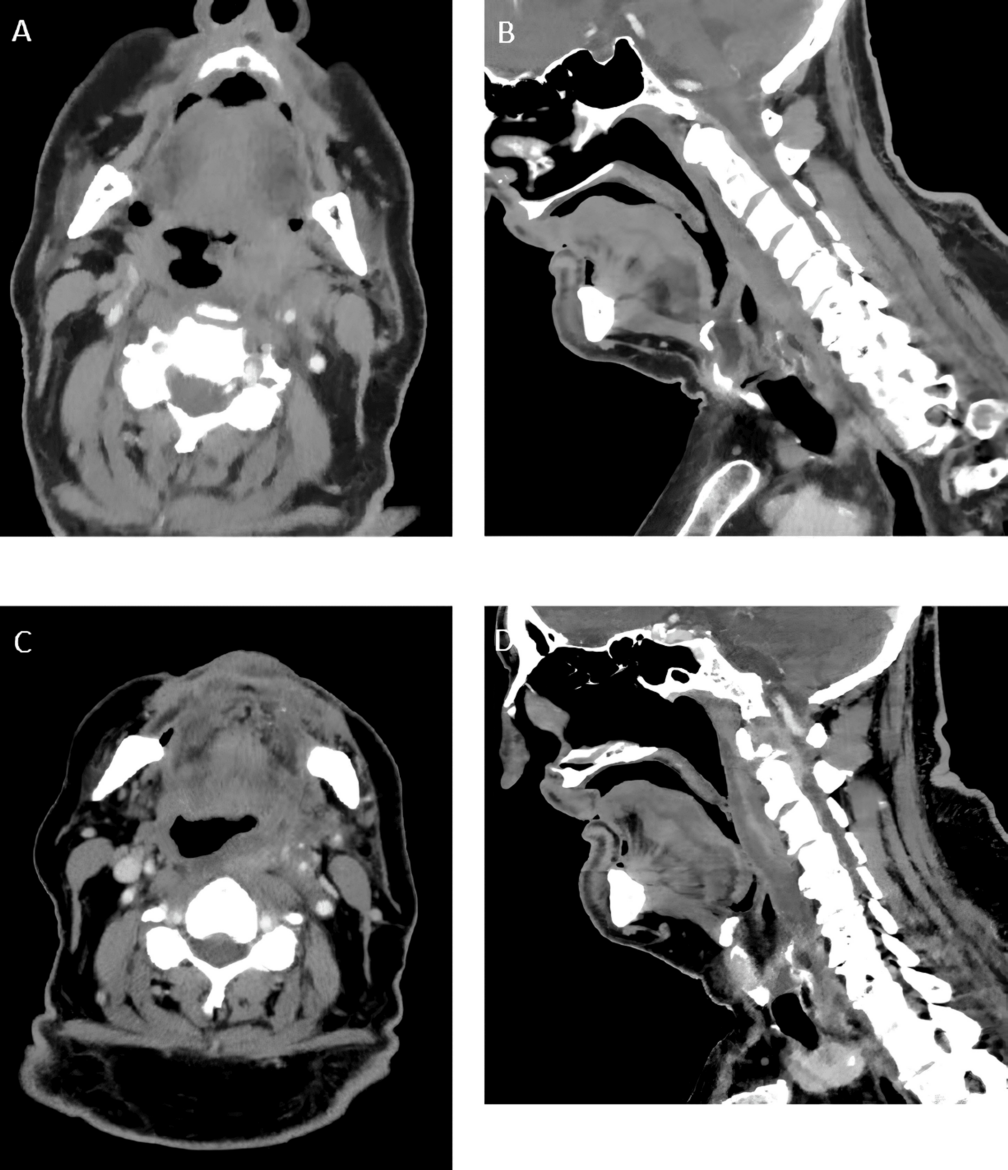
Serial CT images in axial (A, C) and sagittal (B, D) planes demonstrating progressive para- and retropharyngeal effacement. Initial CT (A, B) suggested an infiltrative mass. Subsequent imaging (C, D) demonstrated a more well-defined linear region of density extending from C2 to C4, suggestive of calcific tendinitis of the longus colli. CT, computed tomography
